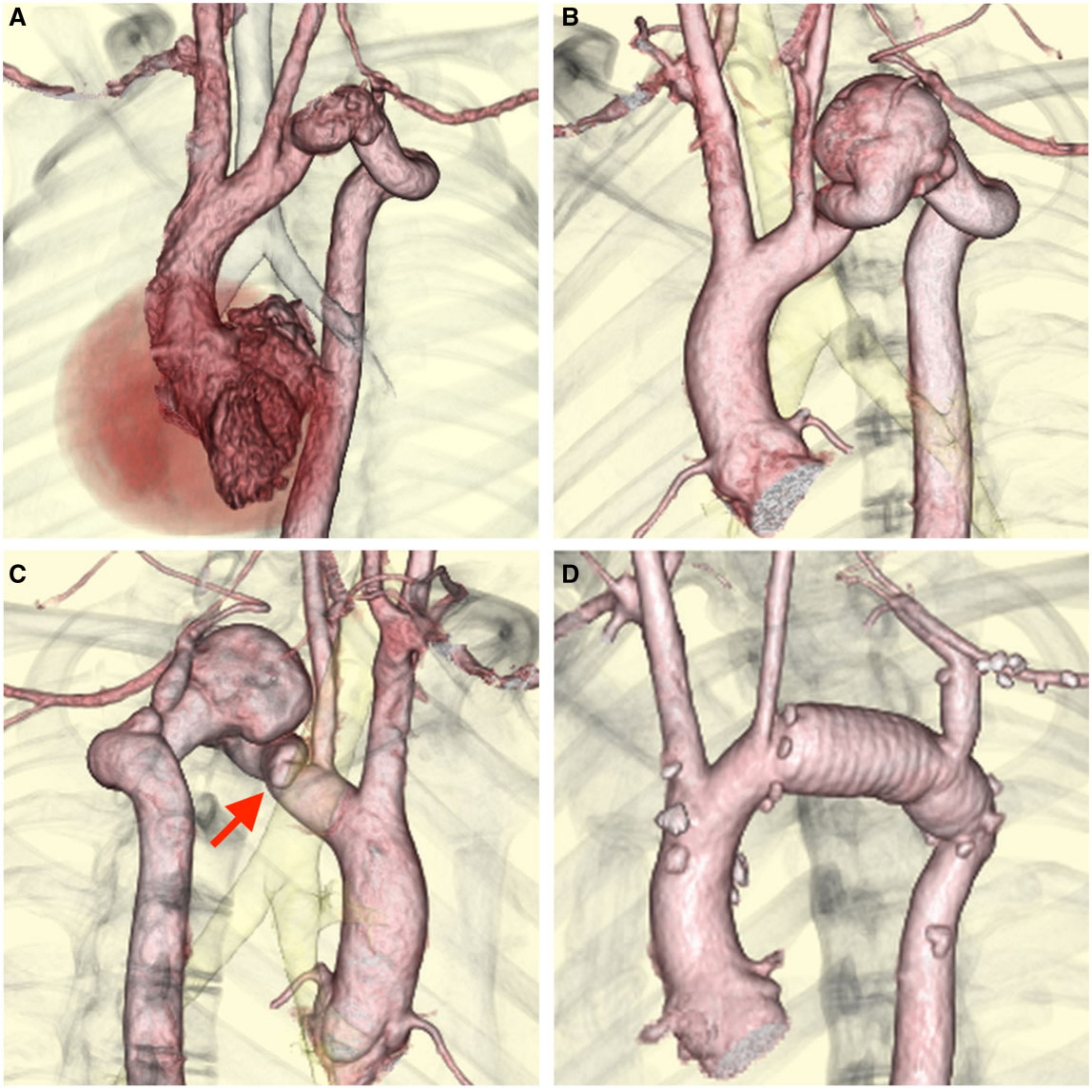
(A) Computed Tomography (CT) angiography at 8 years of age revealed pseudocoarctation of the aorta without aneurysm formation or significant stenosis. (B and C) Preoperative CT angiography revealed saccular aneurysm of the distal aortic arch. Kinking and a tortuous aorta is observed just beneath the left common carotid artery (arrow). (D) Postoperative CT angiography revealed a well-replaced distal aortic arch and reconstructed left subclavian artery.
